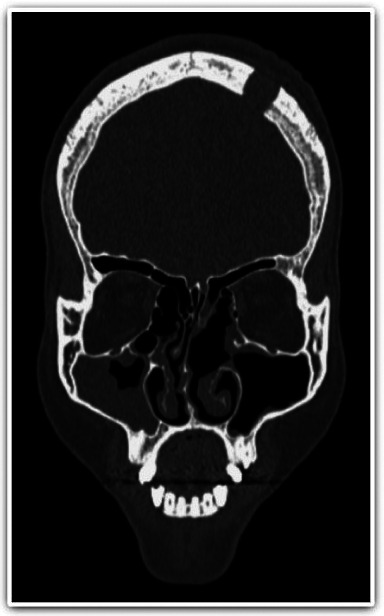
Coronal CT showing resolution of sinusopathy on the operated left side and persistence of sinusopathy on the right side.
